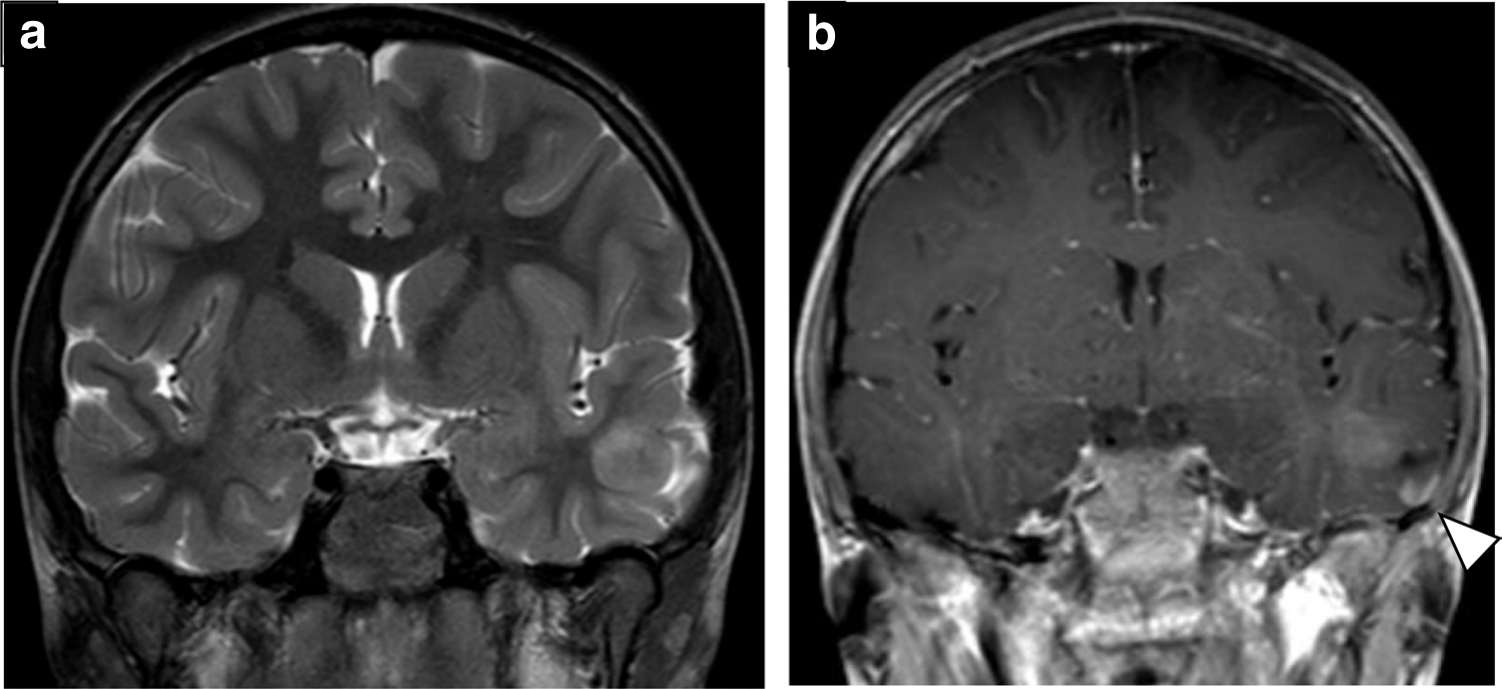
Pleomorphic xanthoastrocytoma (PXA) with *BRAF V600E* mutation and loss of *CDKN2A* in an 8-year-old male who presented with a seizure. Coronal T2-weighted (a) and T1-weighted contrast-enhanced (b) MR images show a solid, mildly expansile and T2-hyperintense mass in the left temporal lobe with faint post-contrast enhancement. Note the more avidly-enhancing focal meningeal thickening and enhancement (*arrowheads*)
